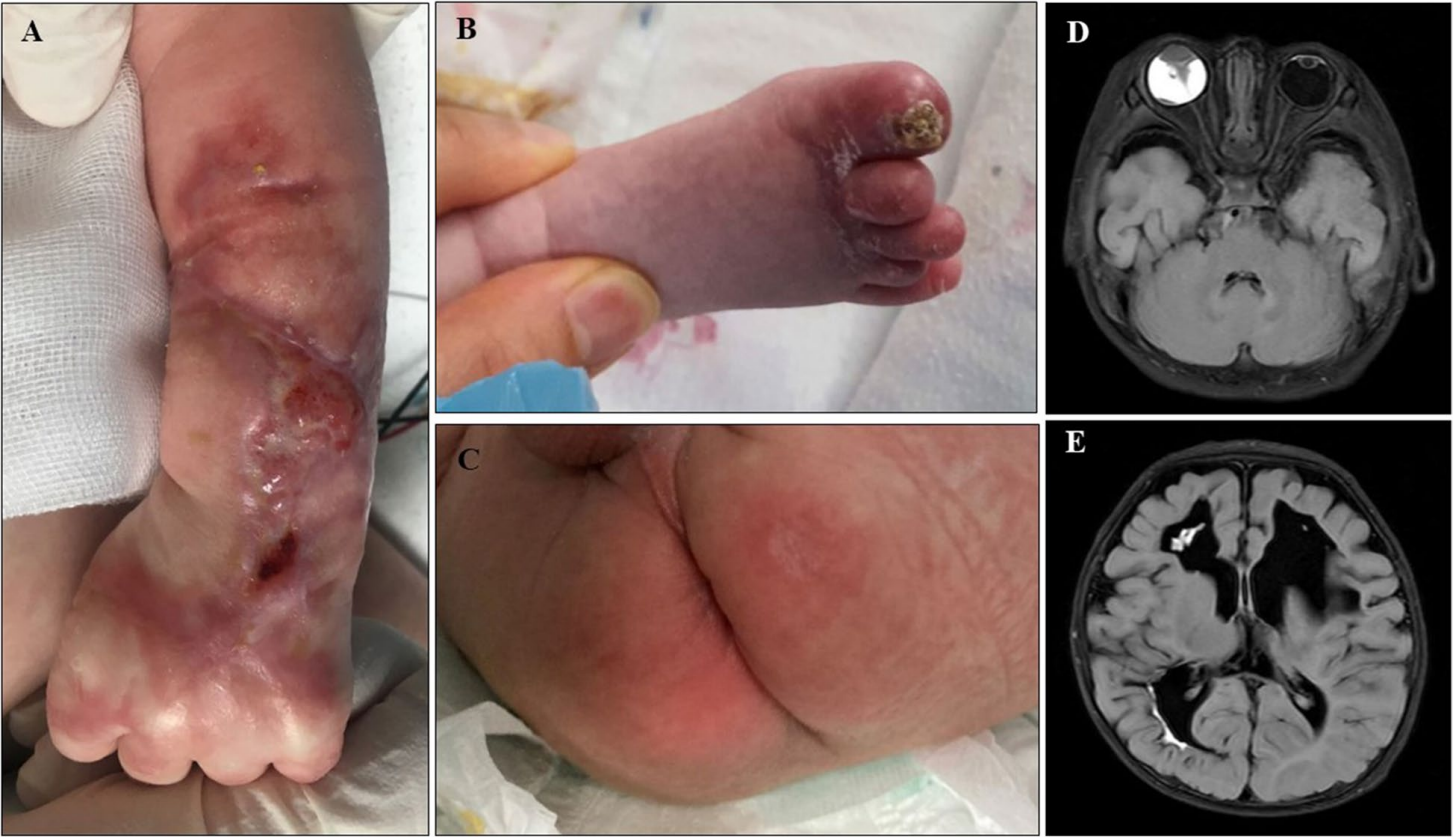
(A–C) Healed lesions at 2 months of age after replacement therapy with protein C concentrate. (D–E) Brain MRI (T2 FLAIR image) obtained at 2 months of age showing remaining diffusion restrictions along previously infarcted areas, suggesting ongoing loss of ischemic tissue
